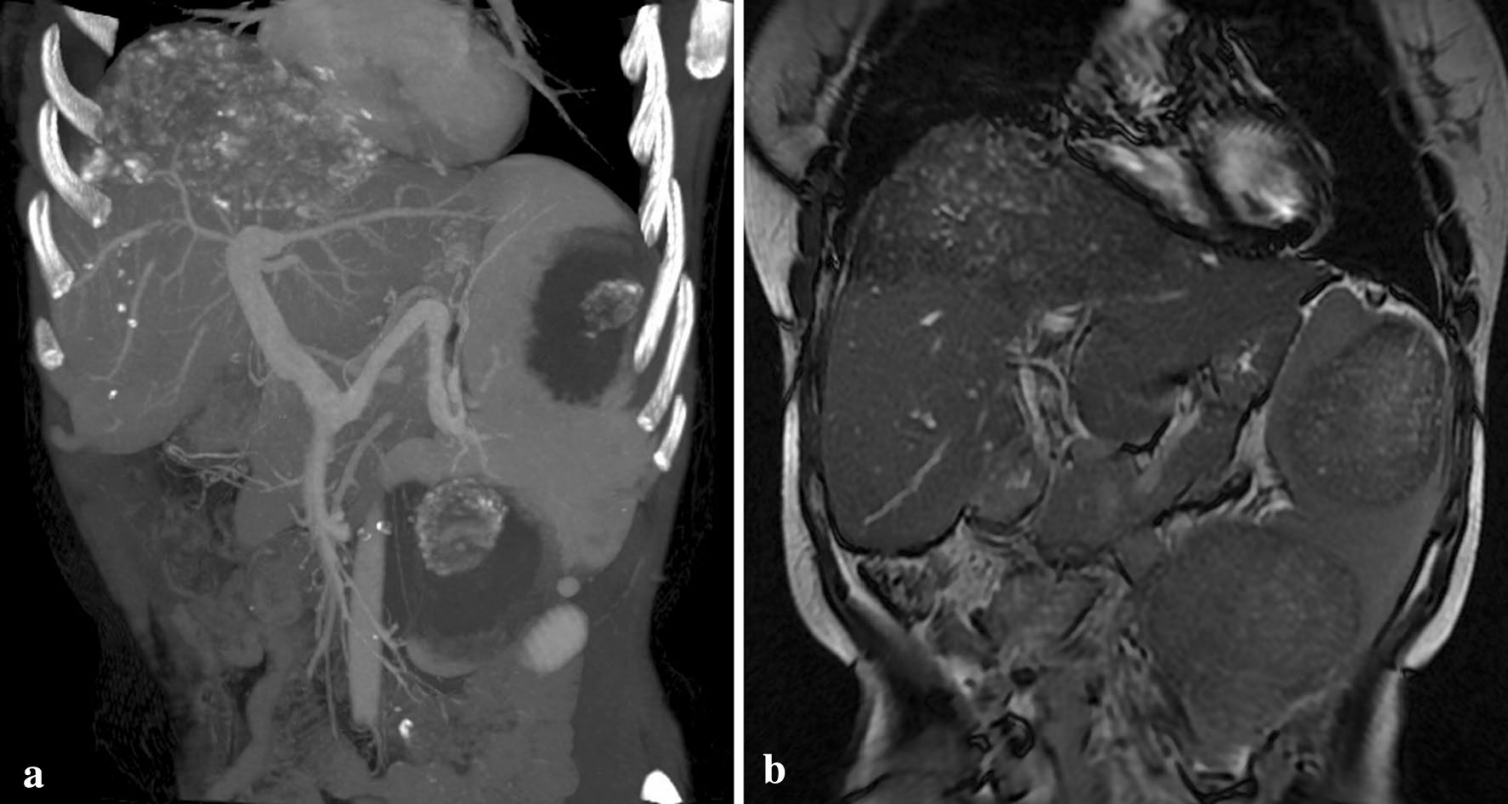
Two lesions were low density with nodular calcification in a coronary MSCT image (a) and heterogeneous intensity in a coronary T2-weighted MR image (b). This case also had hepatic AE.
